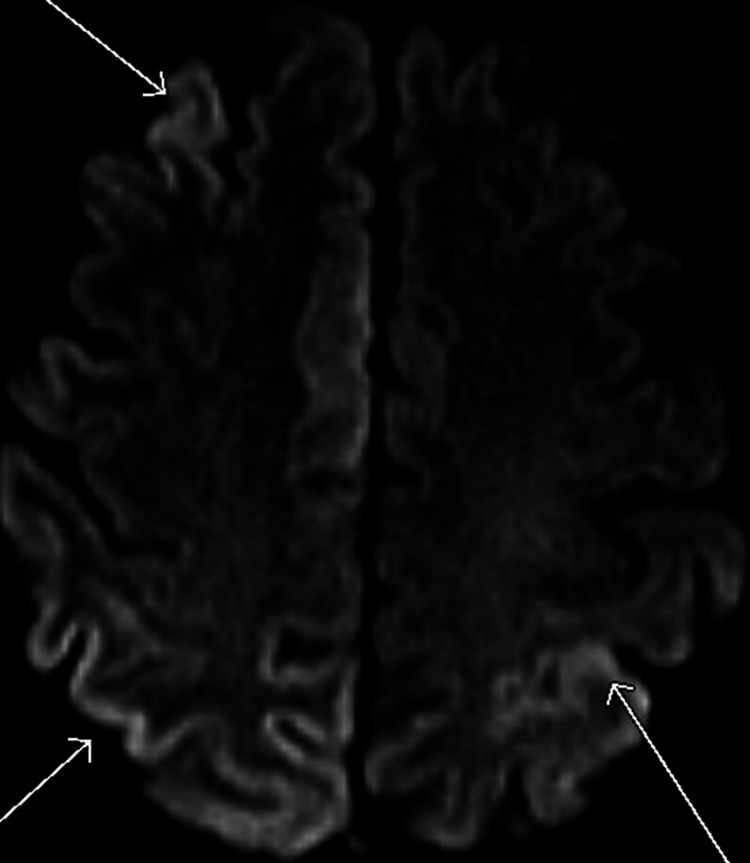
MRI brain showing cortical hyperintensity and ribboning
